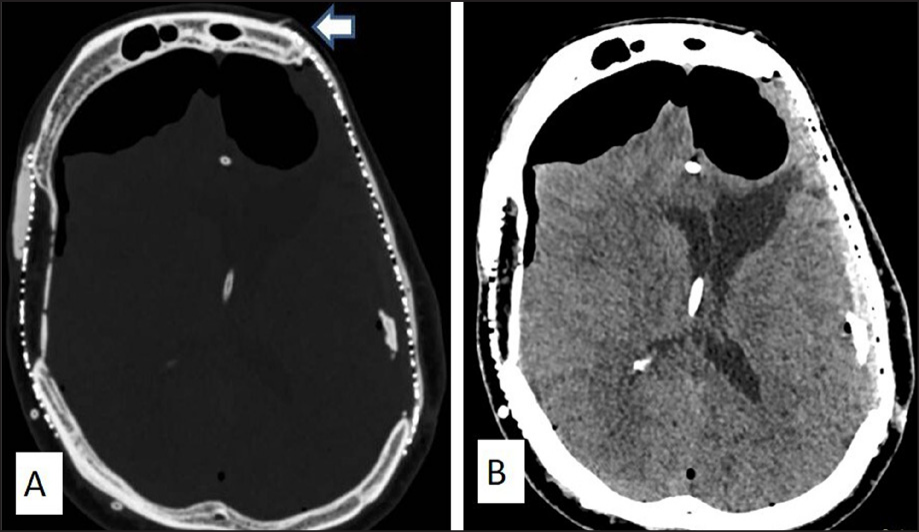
Axial brain CT of a 68-year-old man with bilateral titanium cranioplasty, who developed pneumocephalus five years after surgery due to erosion of the titanium plate through a left frontal scalp wound (A, arrow). Pneumocephalus compressed both frontal lobes resulting in a tented appearance of the brain known as the Mount Fuji sign, indicative of tension pneumocephalus (B). After removal of the titanium plate, the scalp was reconstructed with a rotation flap and skin graft.
